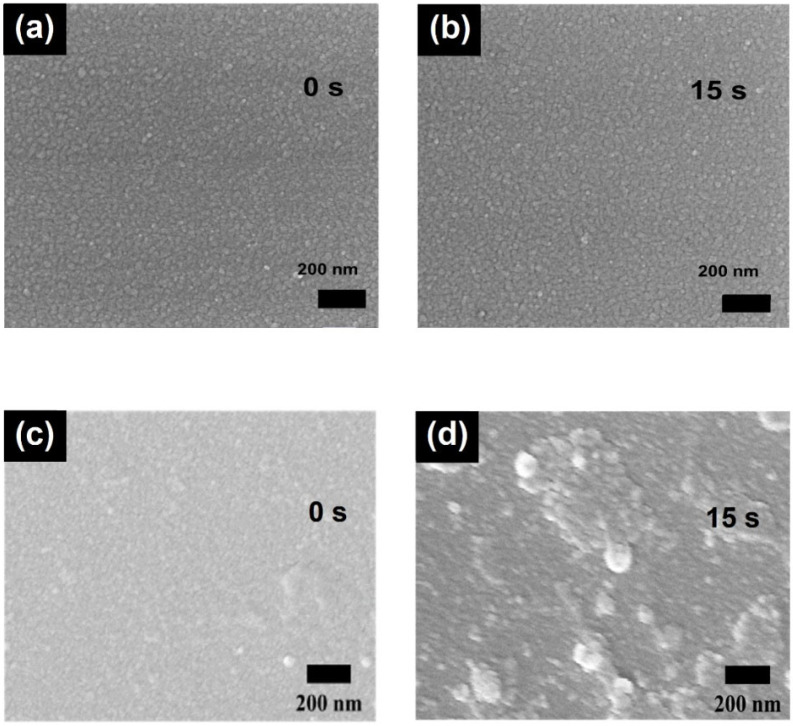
SEM images of the Cr/Al/Cr/TiN anode under the ultrasonic treatment for (a) 0 s and (b) 15 s; SEM images of the Cr/Al/Cr/Mo anode under the ultrasonic treatment for (c) 0 s and (d) 15 s.
